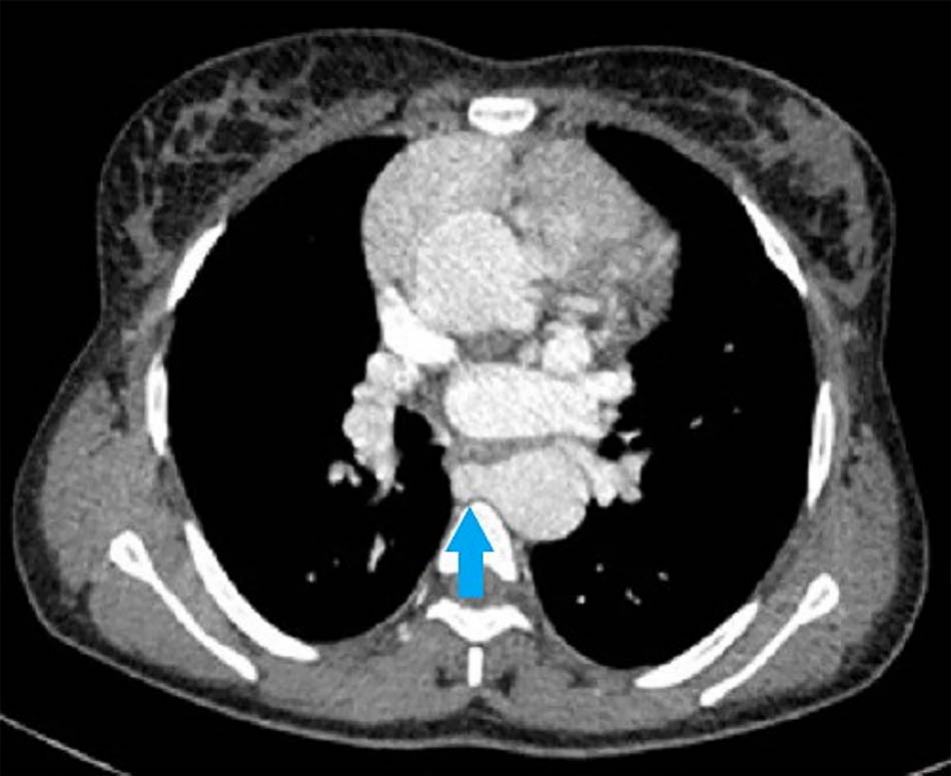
CT chest axial view shows second collateral artery (blue arrow) arising from the right margin of the descending aorta at the level of the left atrium.
